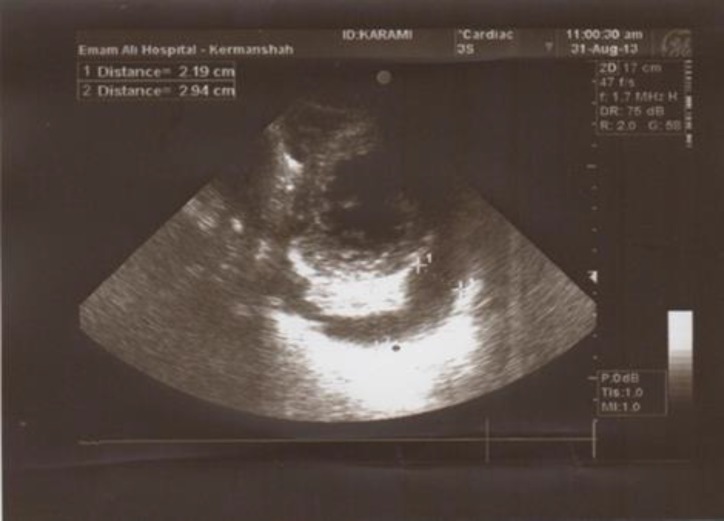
Apical four chamber view shows pericardial effusion
